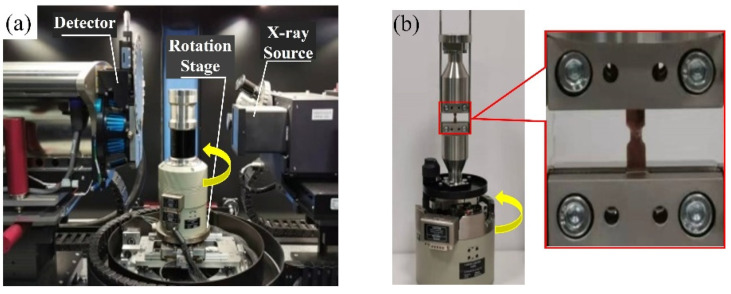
(a) Setup of in situ X-ray μ-CT; (b) tensile setup capable of rotating with μ-CT test piece table.
